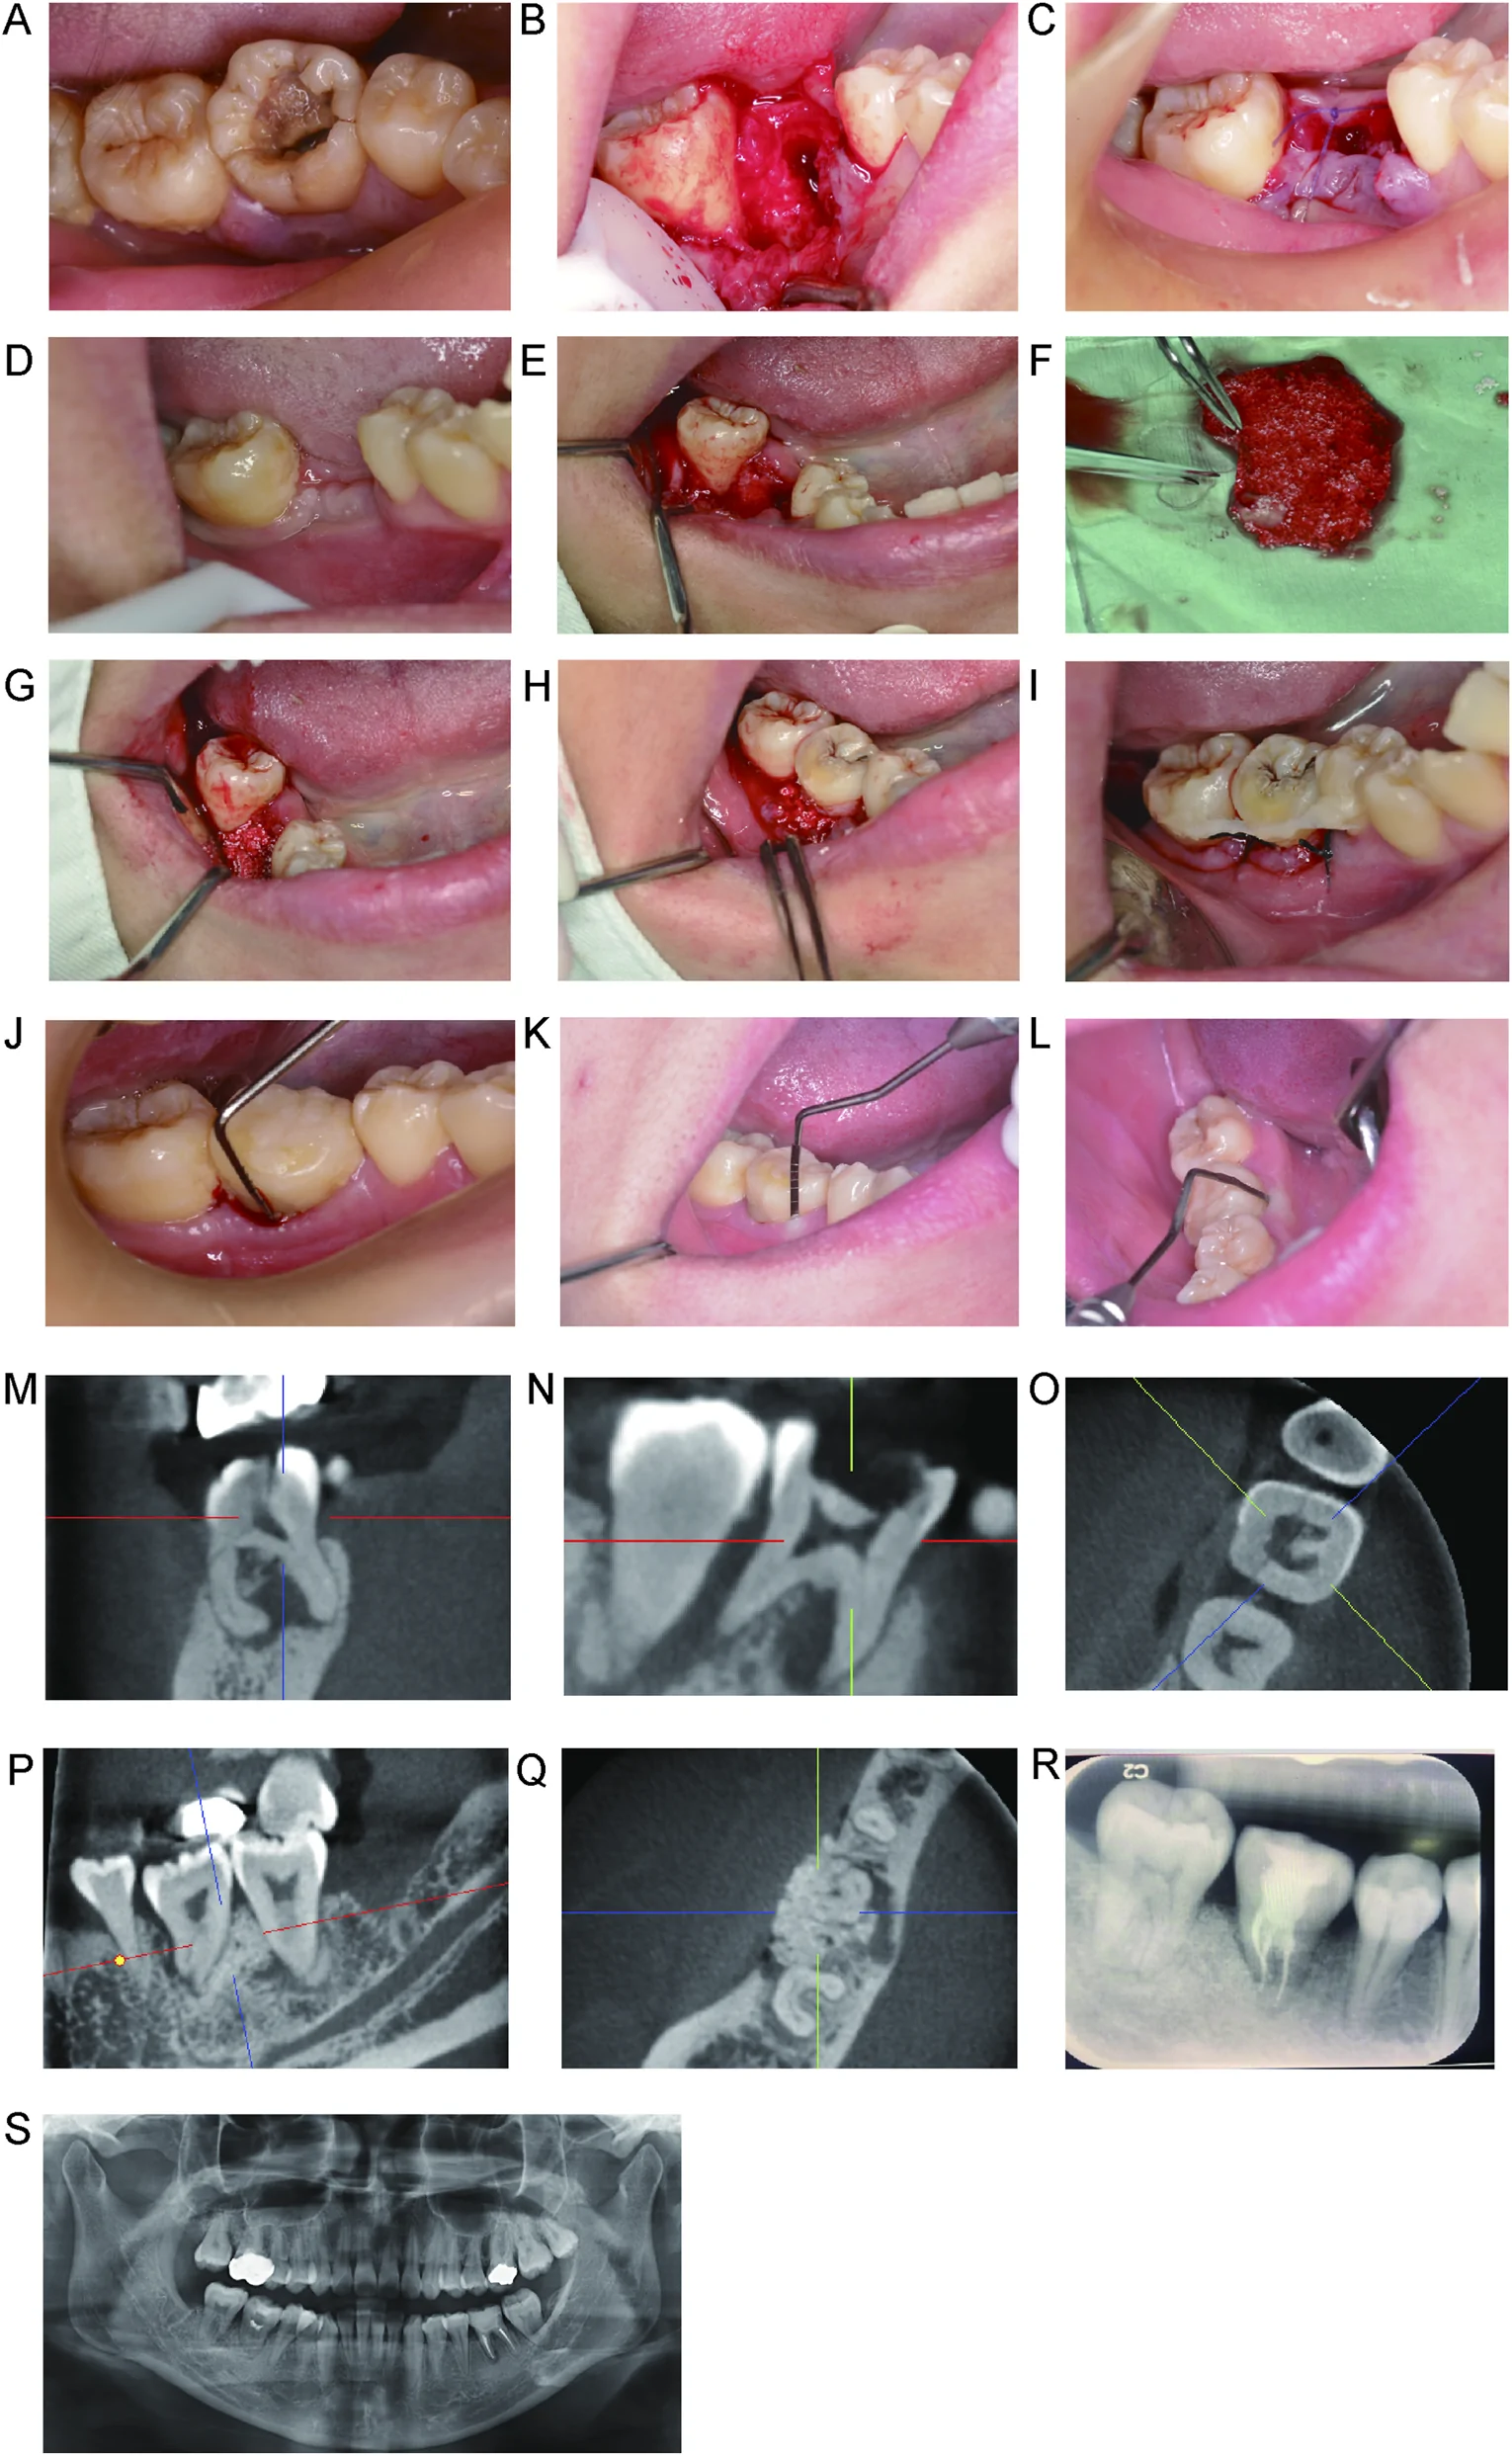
Clinical photographs and radiographic images of Case 1 showing autogenous tooth transplantation of tooth 48 to the tooth 46 recipient site. (A) Initial intraoral photograph of tooth 46 showing extensive crown destruction. (B) Minimally traumatic extraction of tooth 46. (C) Suturing of the extraction socket after removal of tooth 46. (D) Gingival condition at the tooth 46 site 4 weeks after extraction. (E) Flap elevation and preparation of the recipient socket at the tooth 46 site. (F) Preparation of sticky bone. (G) Placement of sticky bone into the recipient socket and alveolar defect at the tooth 46 site. (H) Minimally traumatic extraction of tooth 48 and insertion into the prepared tooth 46 recipient socket. (I) Stabilization of transplanted tooth 48 at the tooth 46 site. (J) Periodontal probing and gingival condition 3 months after autogenous tooth transplantation. (K,L) Periodontal probing and gingival condition at the 1-year follow-up. (M–O) Preoperative CBCT images before autogenous tooth transplantation. (P,Q) Immediate postoperative CBCT image after autogenous tooth transplantation. (R) Periapical radiograph after completion of root canal treatment 3 months after transplantation. (S) Panoramic radiograph at the 1-year follow-up. ATT, autogenous tooth transplantation; CBCT, cone-beam computed tomography.
